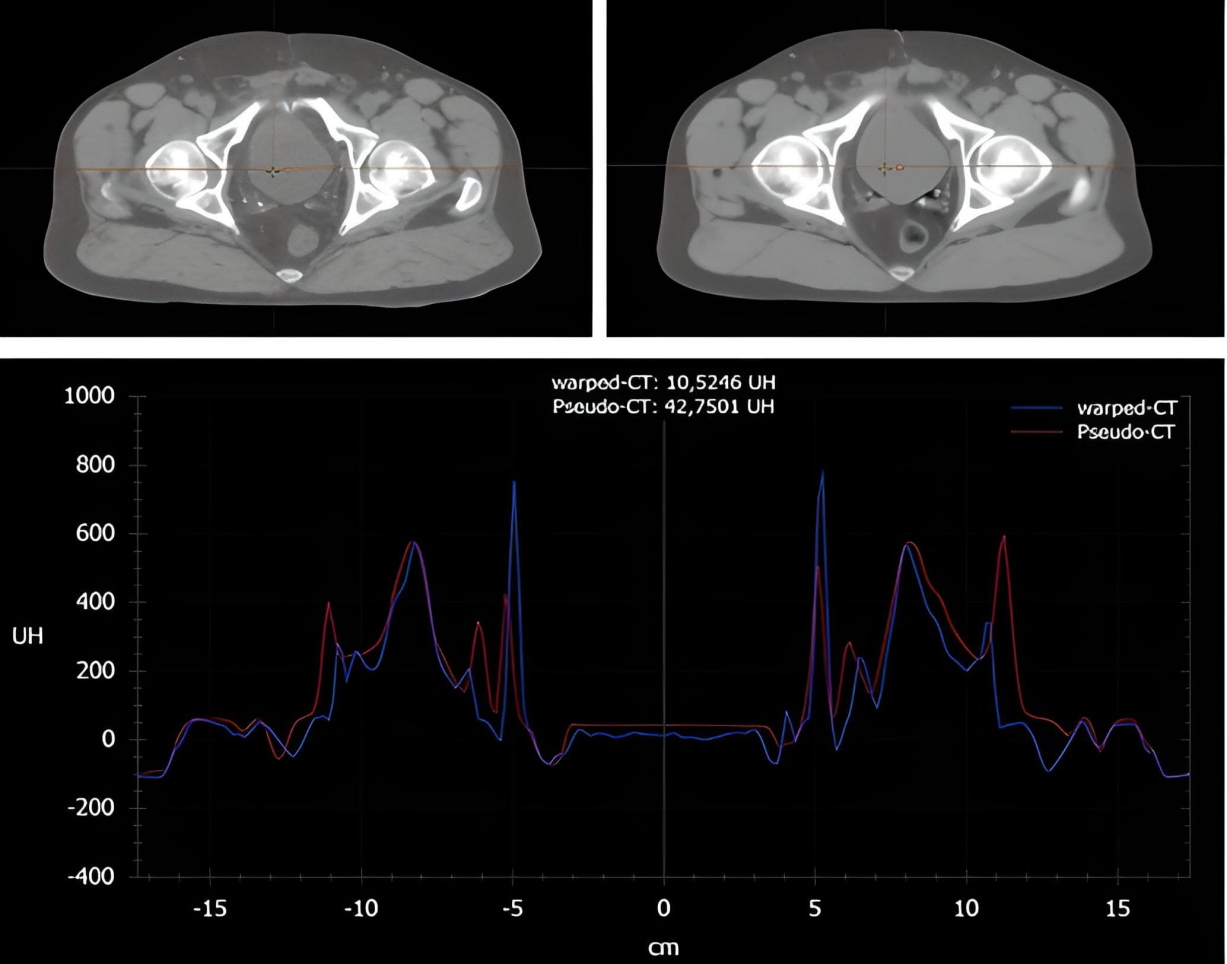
Example of Hounsfield unit comparison between the warped CT (left) and the pseudo-CT (right).
